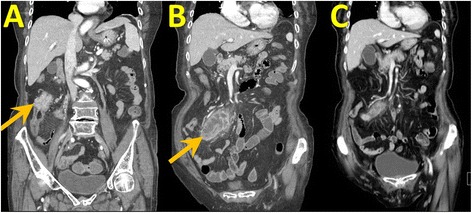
CT scans of the abdomen. An enhanced abdominal CT scan indicated wall thickening of the ascending colon, compatible with colon cancer (a, *arrow*), a swelling appendix with localized abscess, compatible with ruptured appendicitis (b, *arrow*), and regression of appendicular abscess after antibiotics treatment (c)
